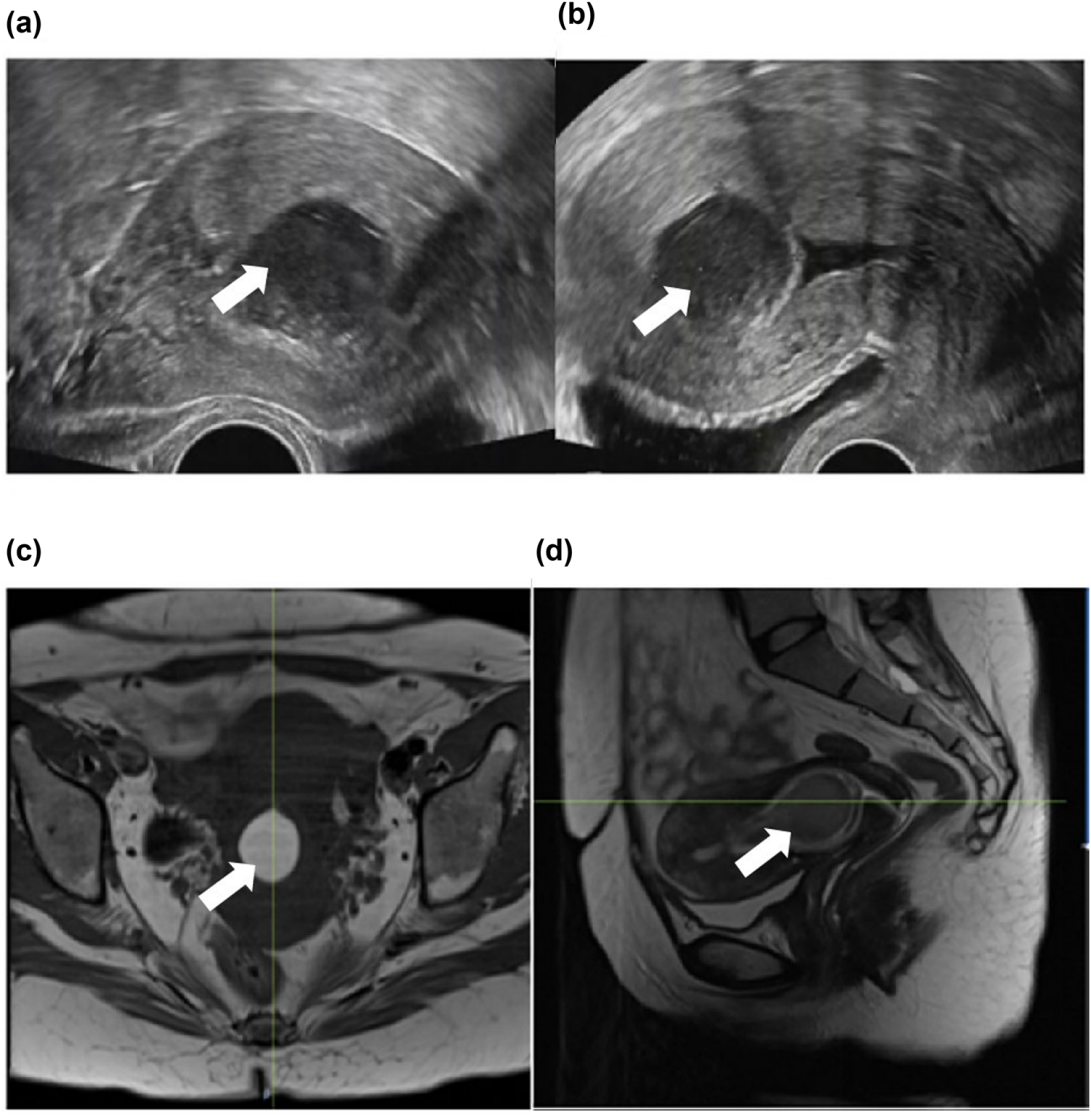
Ultrasonic imaging and MRI of cystic adenomyoma. (a) High signal of lesion in the T1WI cross-sectional images (marked white arrow). (b) Slightly low signal of lesion in the T2WI sagittal images. (c and d) MRI of cystic adenomyoma.
